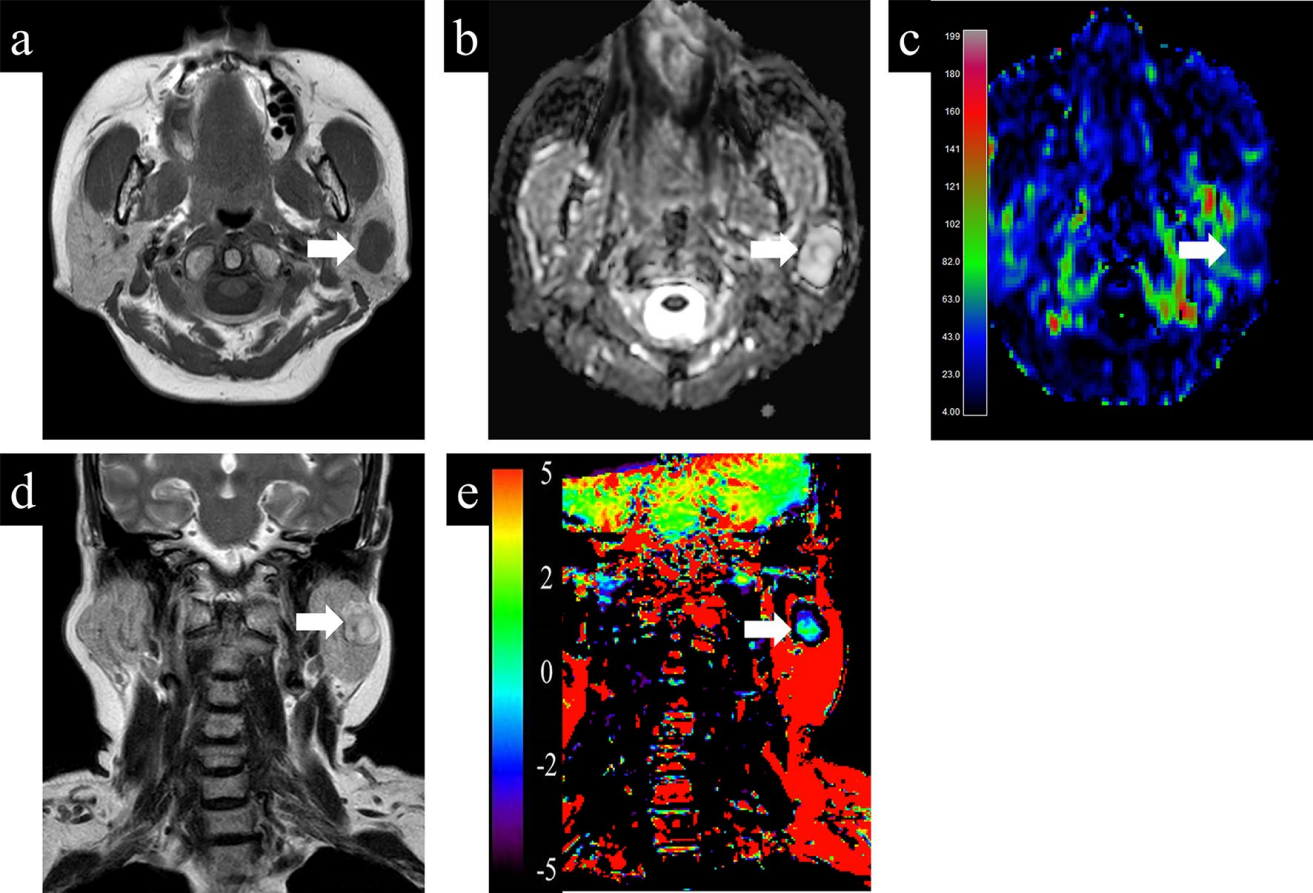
A 68-year-old female with pleomorphic adenoma. (a) T1-weighted image shows a homogeneous low intensity lesion in the left parotid gland. Apparent diffusion coefficient (ADC) (b) and tumor blood flow (TBF) (c) maps show the lesion with the mean ADC value of 1.88 × 10^−3^ mm^2^/s and the mean TBF value of 22.55 mL/100 g/min, respectively. On T2-weighted coronal image (d), the lesion shows higher signal intensity than the adjacent parotid gland parenchyma. Amide proton transfer (APT) (e) map shows the lesion with the mean APT-related signal intensity of 0.26%. An arrow in each image indicates the lesion.
